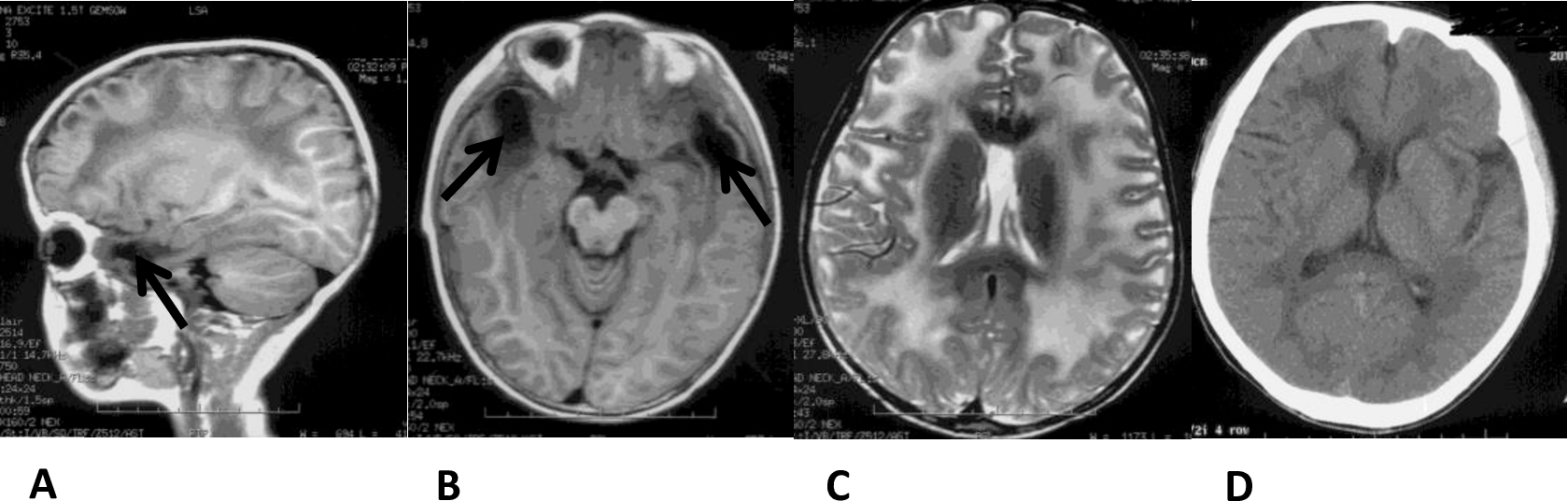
CT and MRI of Pt2. (A-C): cranial MRI at his 4.08yo. Diffuse and abnormal swollen white-matter signals and subcortical cysts in the temporal lobes (arrows); (D) CT at his 12.75yo indicated no changes in the white matter and subcortical cysts.
